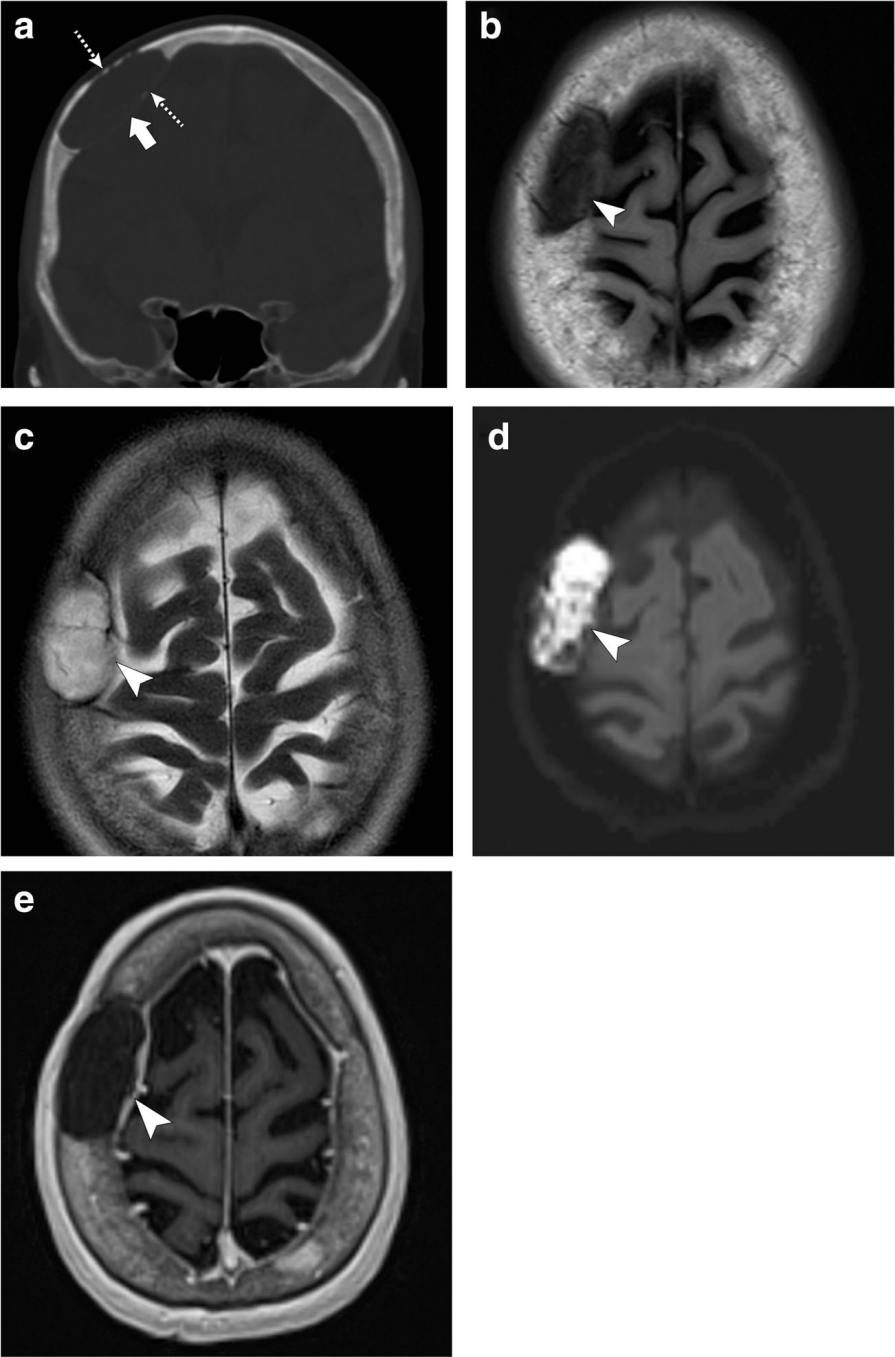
Epidermoid cyst. Axial head CT portrays an expansile lesion in the right frontal bone (*thick arrow*) with thinning of the inner and outer tables (*dashed arrows*). Axial T1-weighted imaging (b), axial T2-weighted imaging (c), axial DWI (d) and axial post contrast T1-weighted imaging (e) show that the lesion is hypointense on T1, hyperintense on T2 with restricted diffusion and absent internal enhancement (*arrowheads*). Note that there may be some enhancement along the peripheral margins
